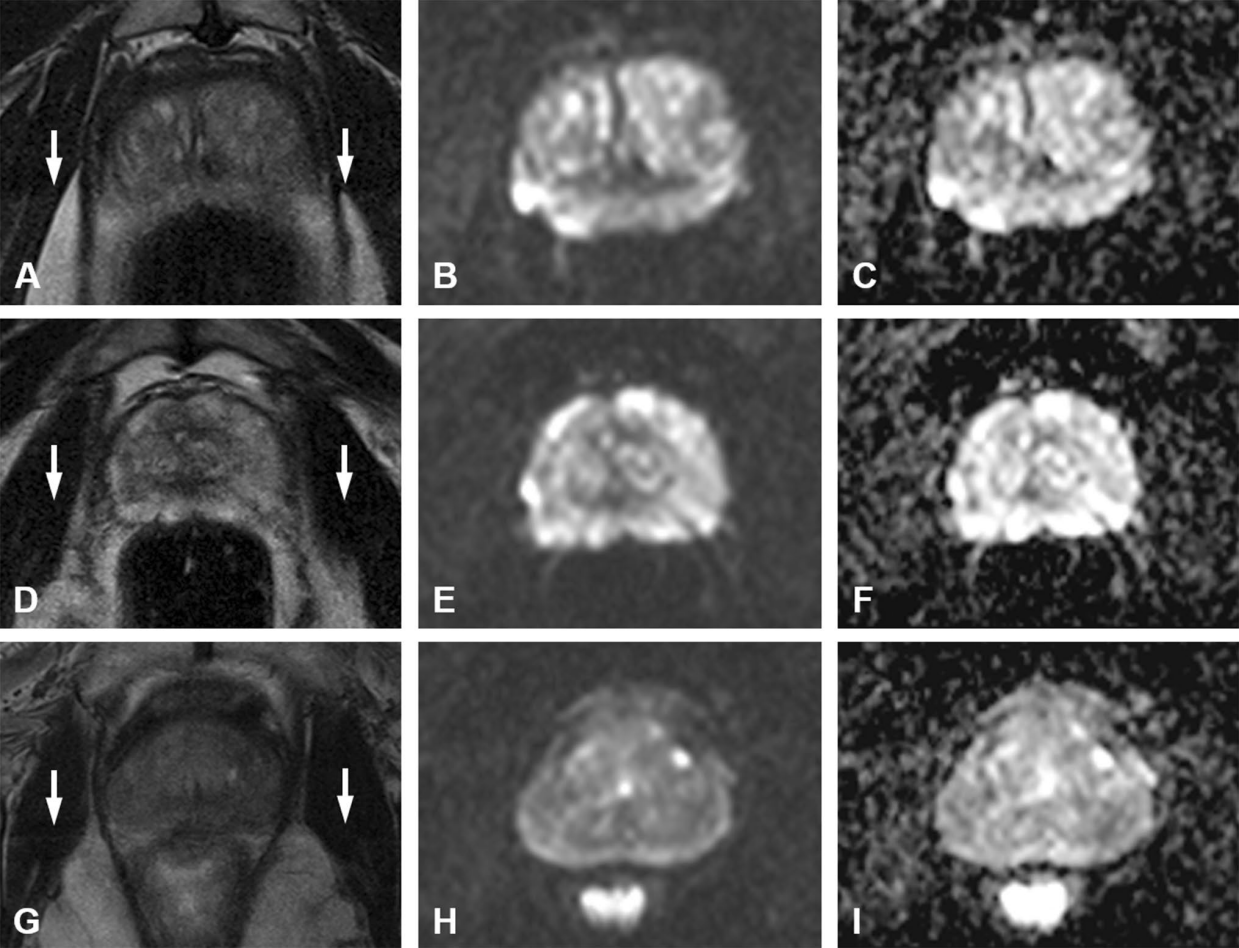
Multiparametric MRI of the prostate of a 63-year old patient (P1) within the cohort HBB−/ME−/DR− (a–c), a 63-year old patient P2 within the cohort HBB+/ME−/DR− (d–f) and a 64-year old patient P3 within the cohort HBB+/ME−/DR+ (g–i). In this setting no patient received microenema (ME). Note the presence of ghosting artifacts (white arrows) on T2w images across all setups- i. e. with and without hyoscine *N*-butylbromide (HBB) and/or dietary restrictions (DR). Moreover, all T2w images are blurred in a similar way
